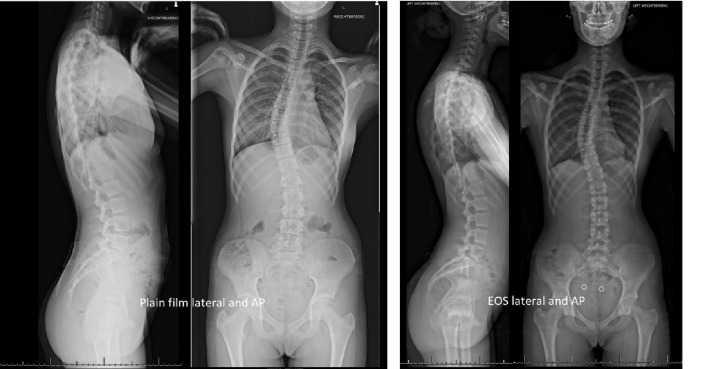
Plain whole spine roentgenogram (left) versus EOS whole spine (right) for qualitative image comparison
